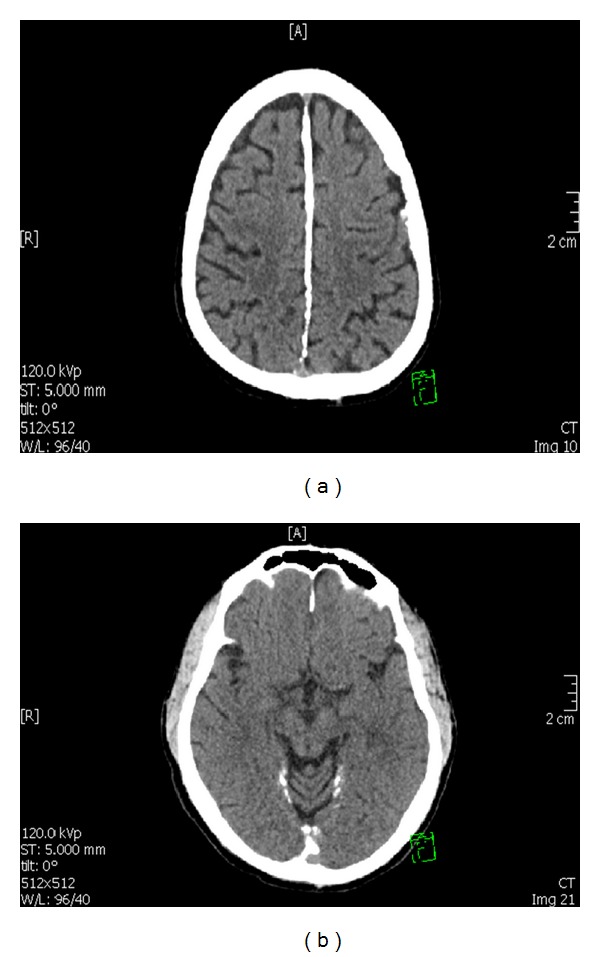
An axial brain CT scan showing extensive brain calcifications along falx cerebri (a) and around the cerebellar vermis with few tiny calcifications in the cerebellum bilaterally (b).
